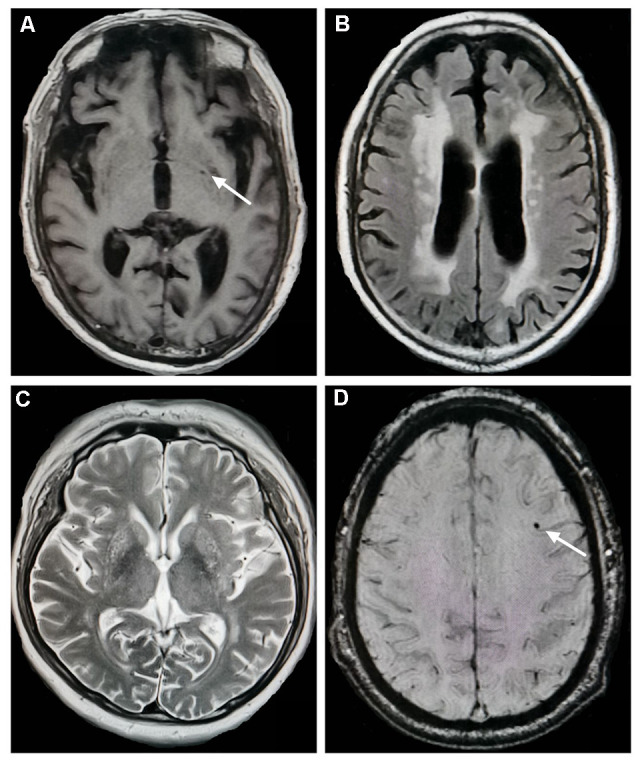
Neuroimaging markers of cerebral small vessel disease. (A) Lacunes (white arrow) in the left basal ganglia (T1-weighted imaging). (B) Severe periventricular white matter hyperintensities (WMH; fluid-attenuated inversion recovery). (C) Enlarged perivascular spaces in the basal ganglia (T2-weighted imaging). (D) Cerebral microbleeds (white arrow) in the left frontal lobe (susceptibility-weighted imaging).
